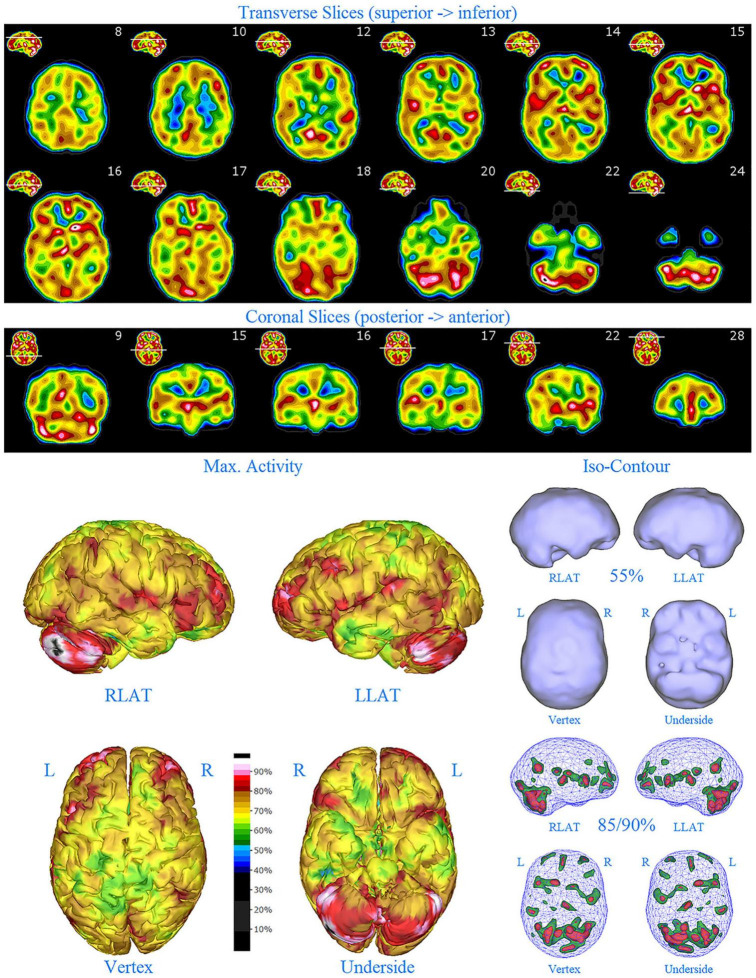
Perfusion SPECT scan of Patient B. Arrangement, color scales, and views are identical to Figure 1. Anatomical structures such as the thalamus are positioned similarly to the scan shown in Figure 2. Labeling was eliminated to avoid obscuring the scan details. Perfusion of the thalamus is increased and asymmetrical. Perfusion is markedly increased in the left caudate. Multiple cortical areas of increased perfusion are evident, predominately in the frontal cortices.
